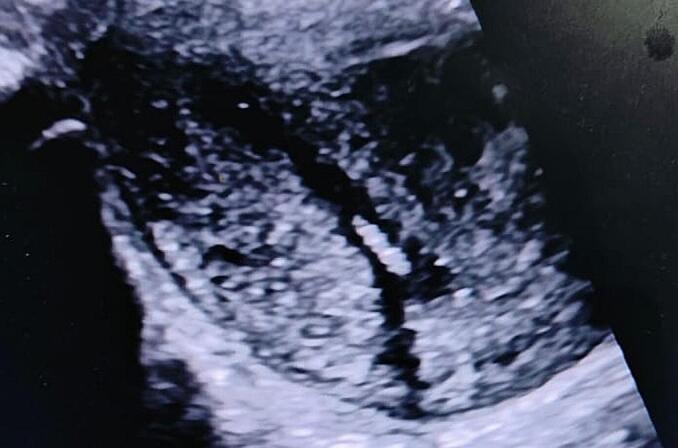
Sonographic image of maggots located low in the uterine cavity.
